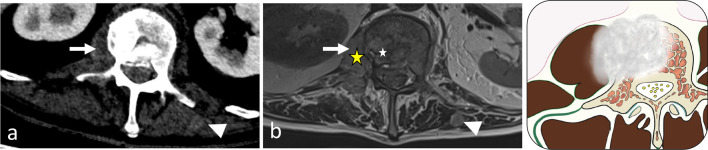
Muscle and bone metastasis* from a lung cancer in a 70-year-old male with acute back pain. Contrast-enhanced axial CT scan reconstruction (a) and axial contrast-enhanced T1WI (b) show a spinal metastasis extending into the psoas muscle and the epaxial region (arrow) and an intramuscular metastasis (arrowhead). The yellow star shows the epicentre of the mass
